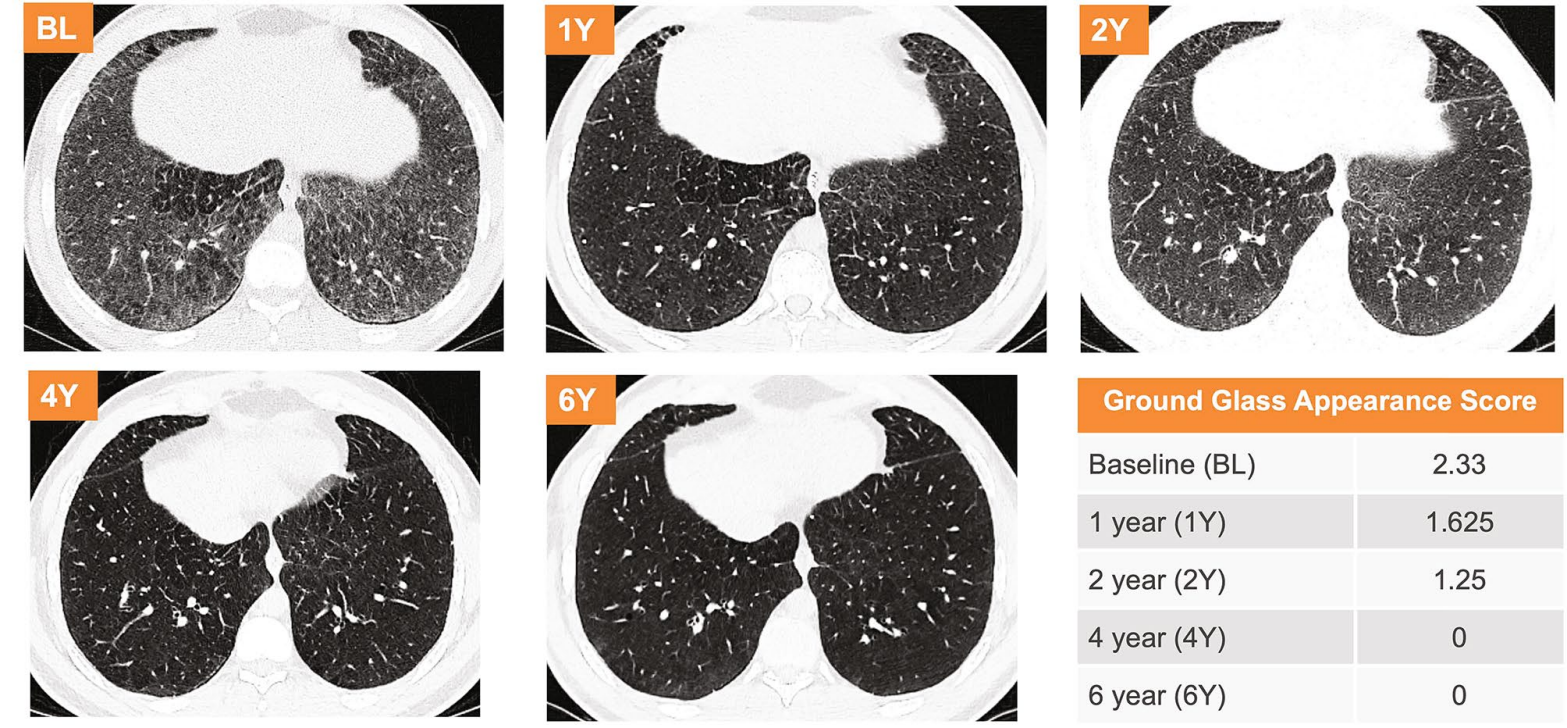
Ground Glass Appearance HRCT Images and Scores. Images and scores at baseline and through year 6 for the individual with the highest (worst) baseline score
